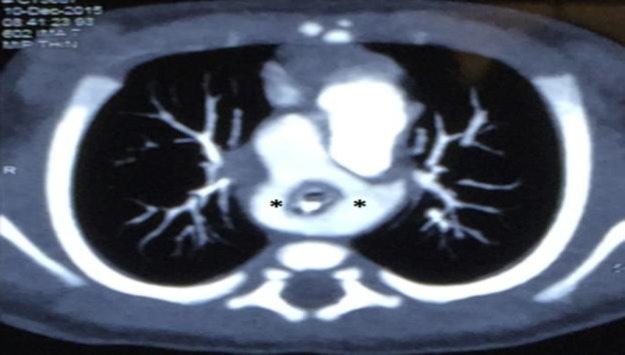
Contrast-enhanced computed tomography (CECT) of the thorax showed both aortic arches form a complete vascular ring, which encasing the trachea and esophagus. Asterisk (*), complete vascular ring
